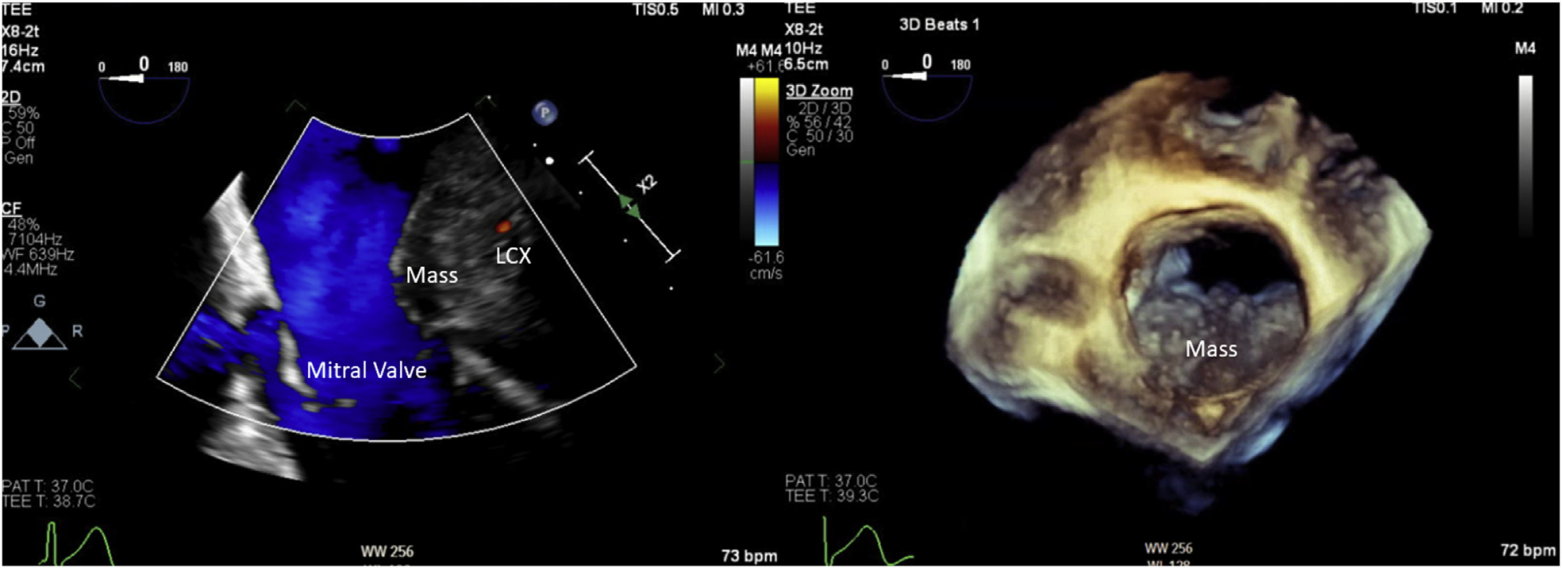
Transesophageal Echocardiography Showing the Mass in the Lateral Left Atrial Wall and Atrioventricular Groove Encasing the Left Circumflex Artery LCX = left circumflex.
